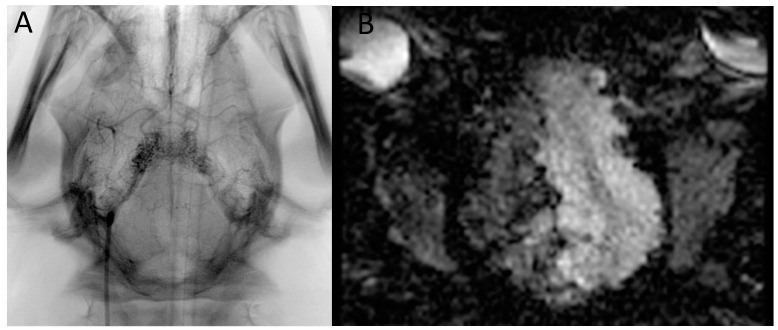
Test infusions through Apollo microcatheter under (A) X-ray and (B) real-time dynamic EPI-GRE MRI monitoring—dynamic images available in the Supplementary Material Video S1.
